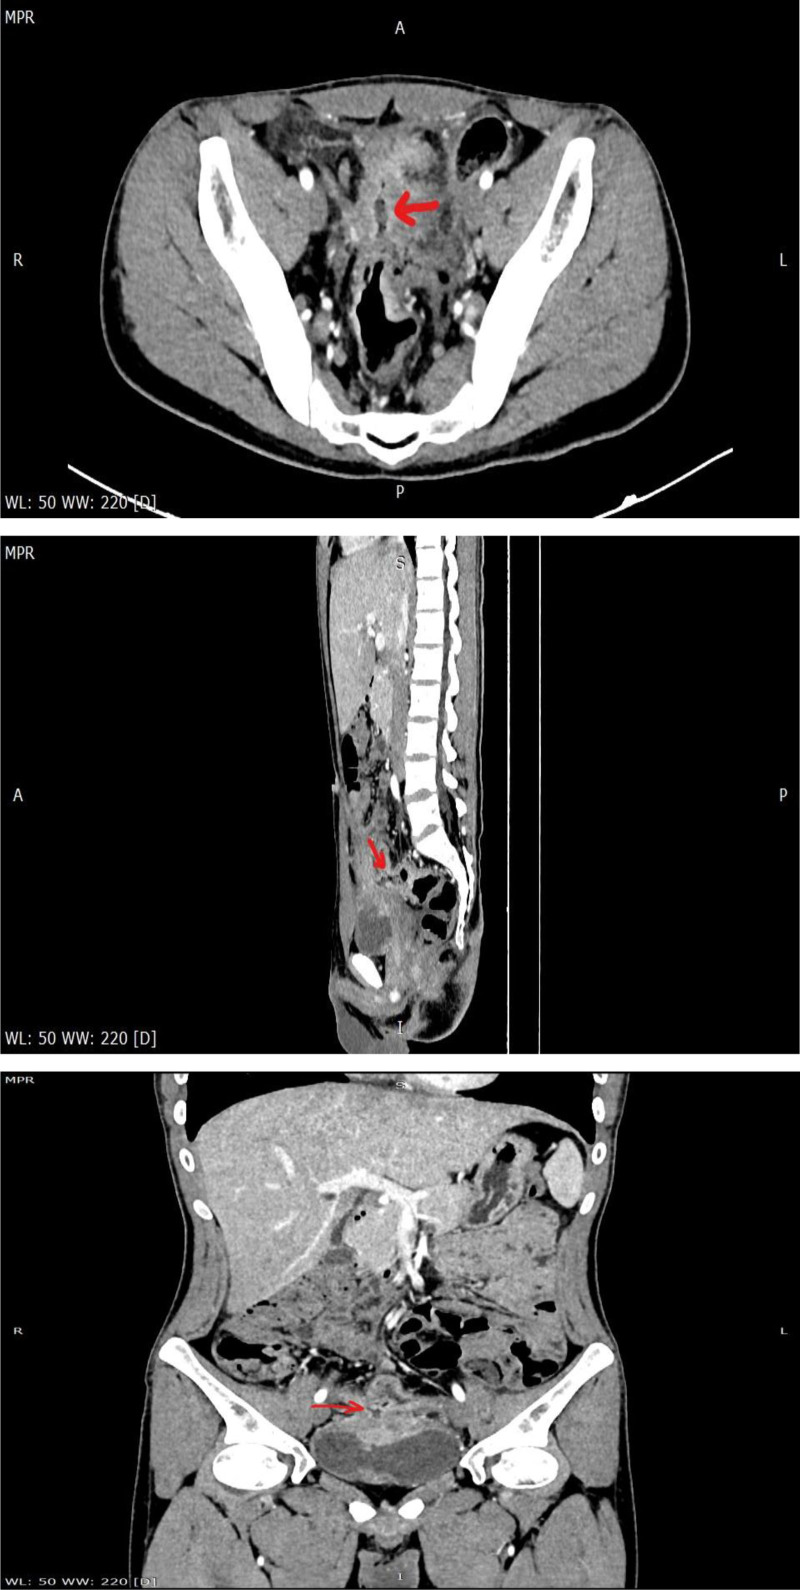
CT manifestations of the small bowel in the diagnosis of Crohn’s disease combined with bladder fistula. CT = computed tomography.
